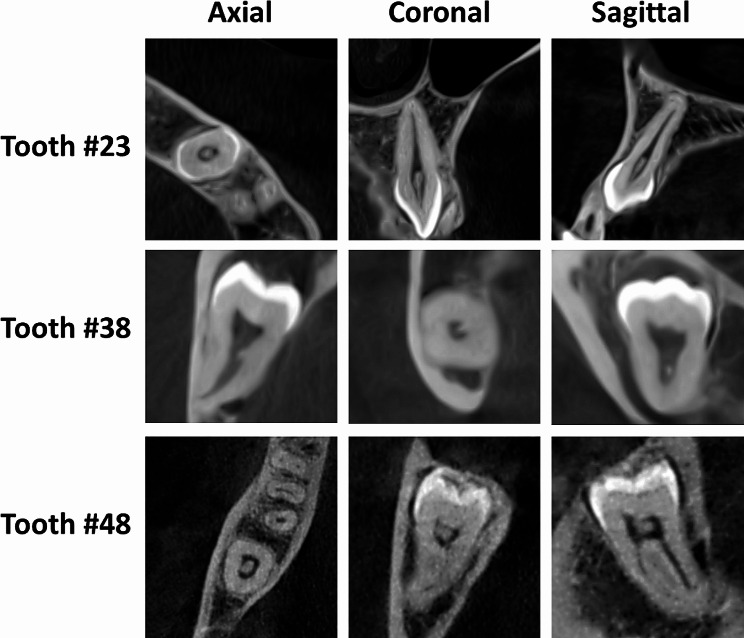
CBCT images showing dental pulp stones in different positions of different unerupted teeth. Teeth #23 and #48 are vertically impacted, whereas tooth #38 is horizontally impacted
